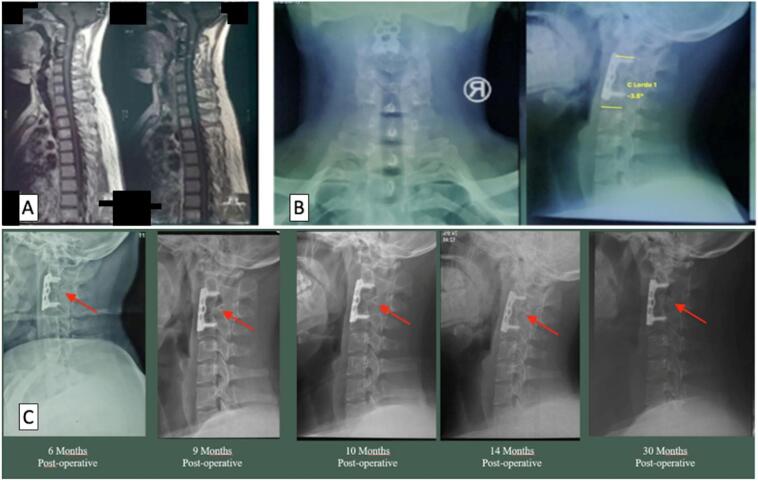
(A) Pre-operative cervical MRI; (B) Post-operative anteroposterior and lateral view cervical X-ray using ACCF on vertebrae cervical 3 with autologous iliac bone graft (red arrow); (C) Consecutive lateral cervical X-ray from 6 months post-operative to 30 months post-operative. Cervical fusion is observable in the last 6 months post-operative. Show fusion process on vertebrae cervical 3 with the implant remained intact and no failure was observed (red arrow). (For interpretation of the references to color in this figure legend, the reader is referred to the web version of this article.)
